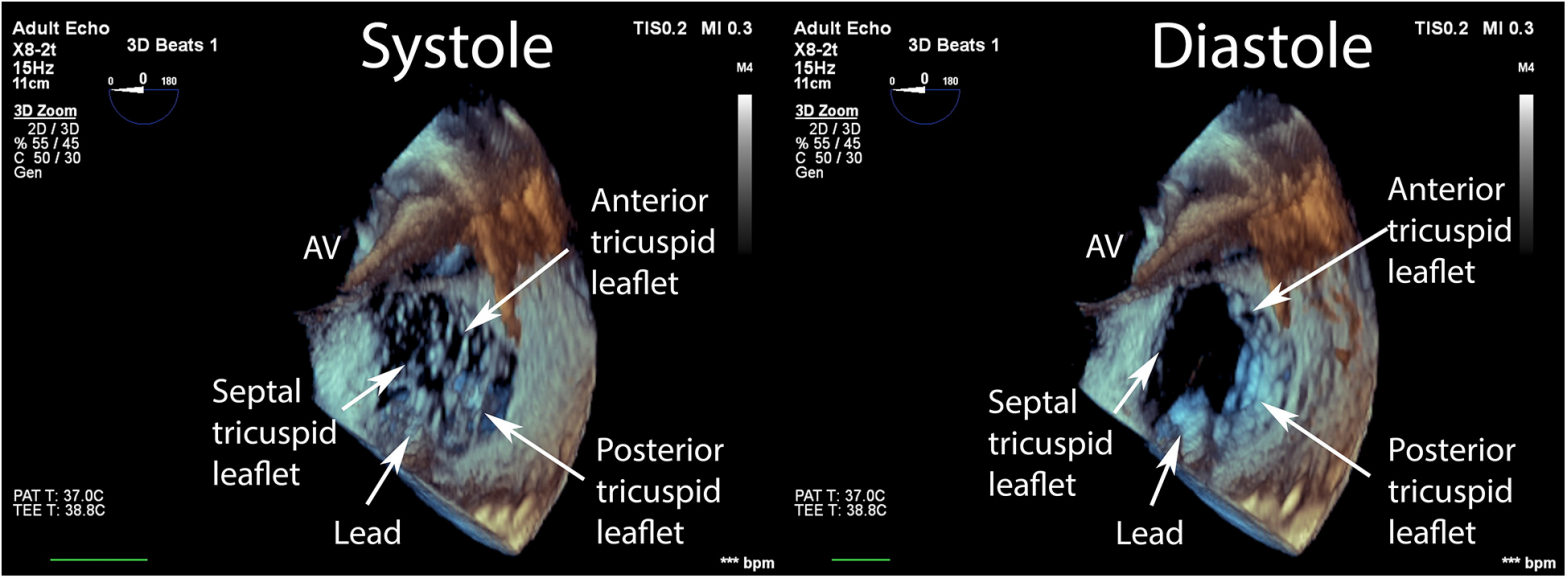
En face* view of the tricuspid valve in real-time, three-dimensional transesophageal echocardiography. Visualization of sufficient quality for transcatheter tricuspid valve repair guidance includes the components of the valve: (i) annulus; (ii) septal, anterior, posterior leaflets; (iii) fragments of the subvalvular apparatus and anatomical landmarks: (iv) fragment of the aortic valve annulus, (v) fragment of the intra-atrial septum, and (vi) vena cava superior.
